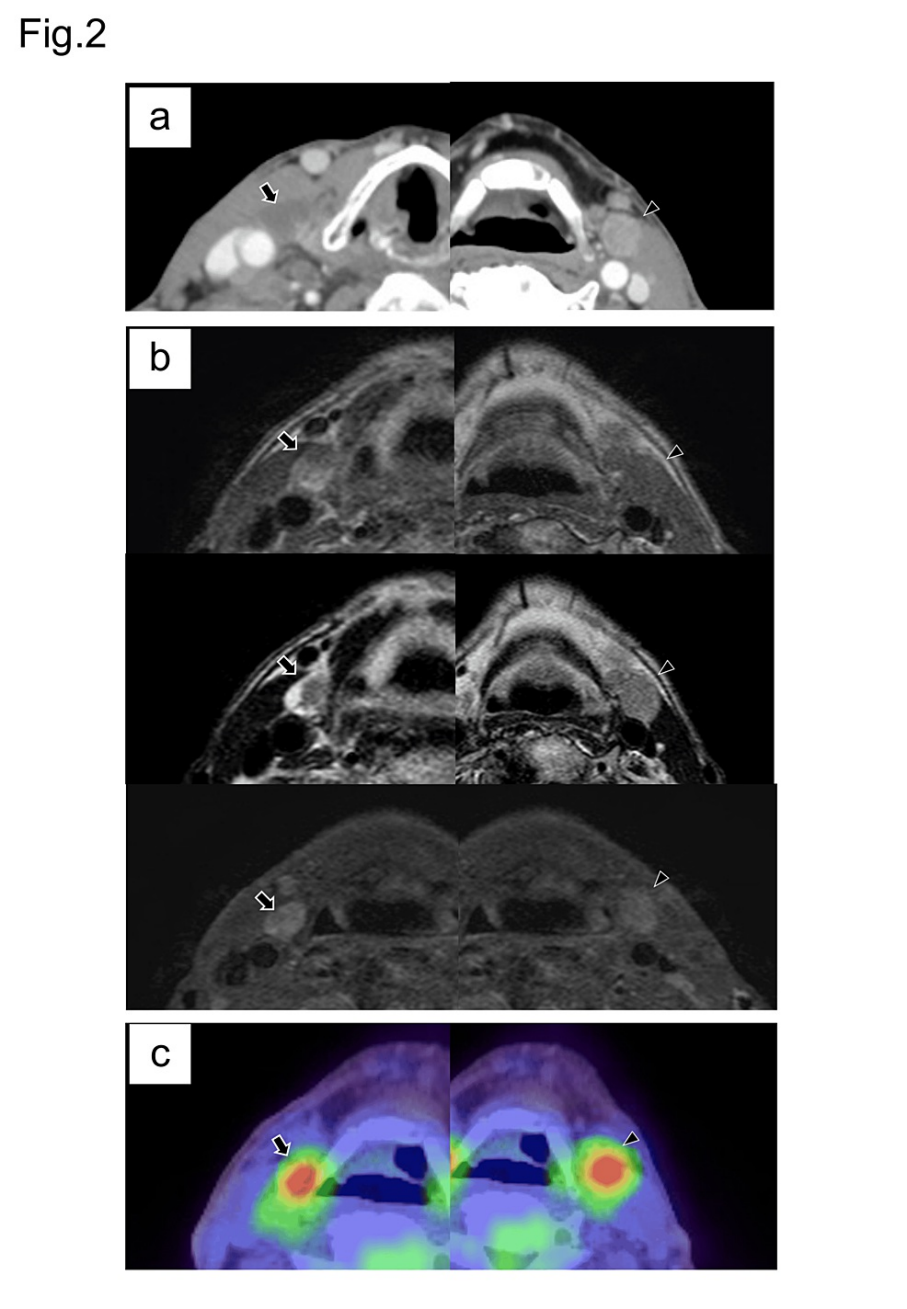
A comparison of imaging findings of the left and right lymph nodes a) Computed tomography showed a solid lymph node in the left neck (arrowhead) and cystic lymph nodes in the right neck (shown with arrows). b) Magnetic resonance imaging showed a focal hyperintense area in the T1-weighted images and a markedly hyperintense area in the T2-weighted images in the periphery of the right cervical node (shown with arrows). The left cervical nodes appeared as solid nodes with nonspecific signal intensity (indicated by arrowheads). The degree of contrast enhancement of the lymph nodes was greater in the right neck than in the left neck. c) Positron emission tomography/computed tomography showed no significant difference in fluorine-18 deoxyglucose accumulation between the right cervical nodes (shown with arrows) and the left cervical node (arrowhead).
